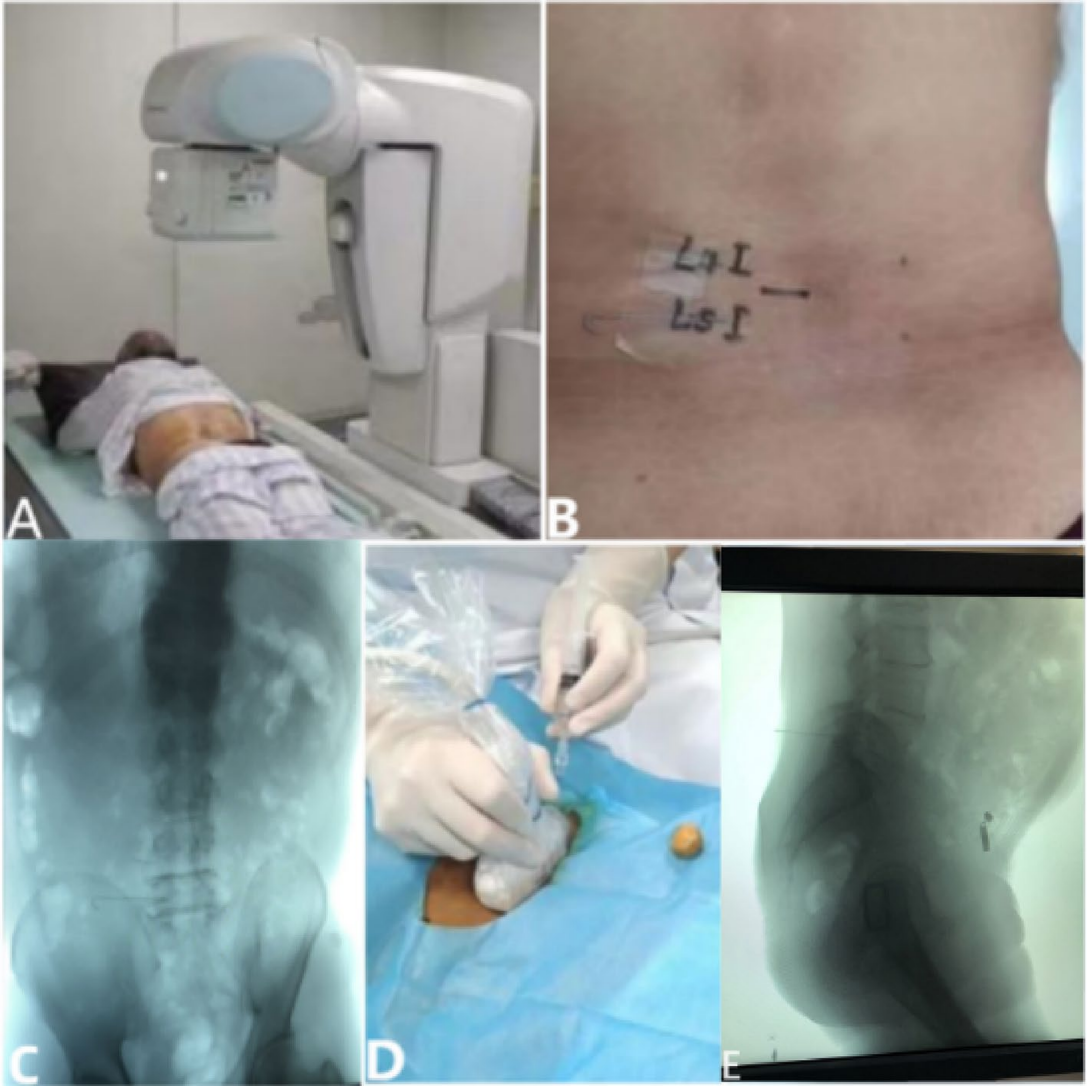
X-ray verification picture: (A) the ultrasound equipment was placed in the X-ray examination room. The patient lies prone on the X-ray bed and the clothes are pulled up to expose the waist and back. (B) The planned block segments are marked on the body surface, and an X-ray marker is attached. (C) X-ray shows that the marker is in the correct position. (D) The block needle was placed under the guidance of ultrasound. (E) X-ray verification: the block needle is accurately in place.
